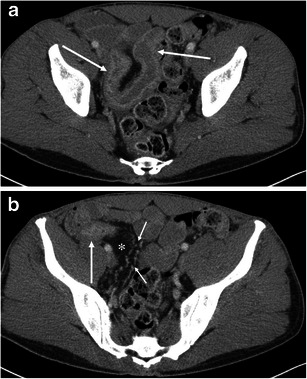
A 42-year-old male with Crohn’s disease. a The axial CE-CT shows wall thickening and hyperenhancement of the terminal ileum (*arrows*). The layer of wall enhancement is thick and irregular. b The terminal ileum near the ileocecal valve shows wall enhancement and luminal narrowing (*arrow*). Creeping fat (*asterisk*) and engorgement of the vasa recta (*small arrows*) are noted
